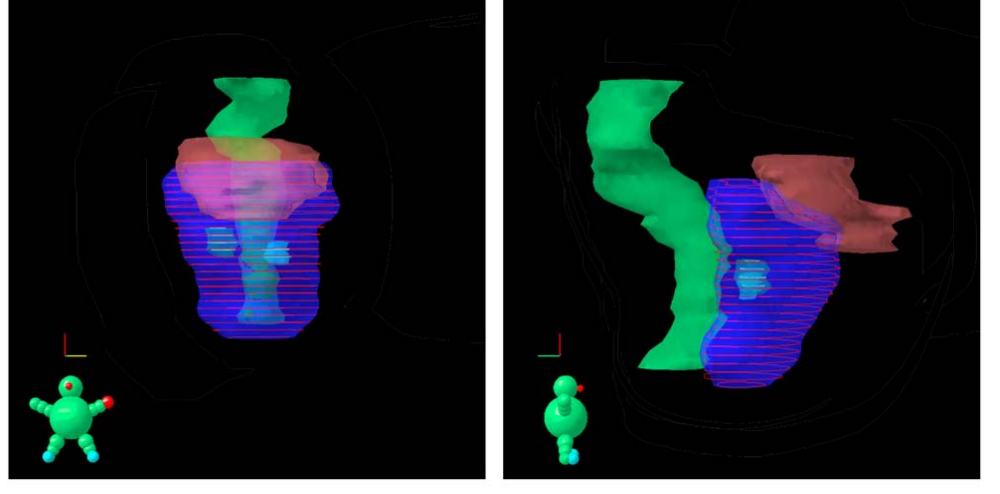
Radiation dose plan showing anterior view on the left panel and lateral view on the right panel. This 56 year old patient had 2 areas on prostate MRI suspicious for cancer. MR guided biopsies of these suspicious areas were performed. Both suspicious areas were positive for Gleason Score 6 prostate cancer. The 94.5 Gy dose clouds of the simultaneous integrated boost are seen in the left and right mid gland as yellow rings around the contoured MR abnormalities of biopsy proven cancer. The 75.6 Gy dose cloud covering the remainder of the prostate is represented by red rings. The rectum (green) and bladder (light brown) are also shown.
